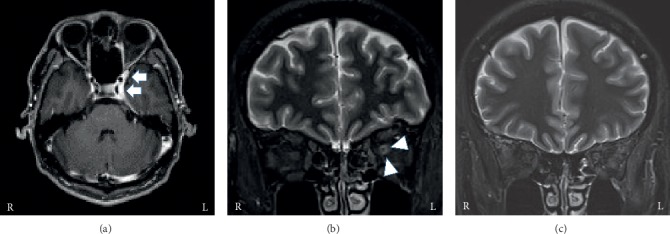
Brain MRI of case 1. (a) The lesion (arrows) is enhanced on T1-weighted image and extends into the superior orbital fissure. (b) The high-intensity ring appearance (arrowheads) around the left optic nerve is seen on short tau inversion recovery (STIR) MRI. (c) The high-intensity ring appearance has disappeared on day 21 after initiating treatment.
